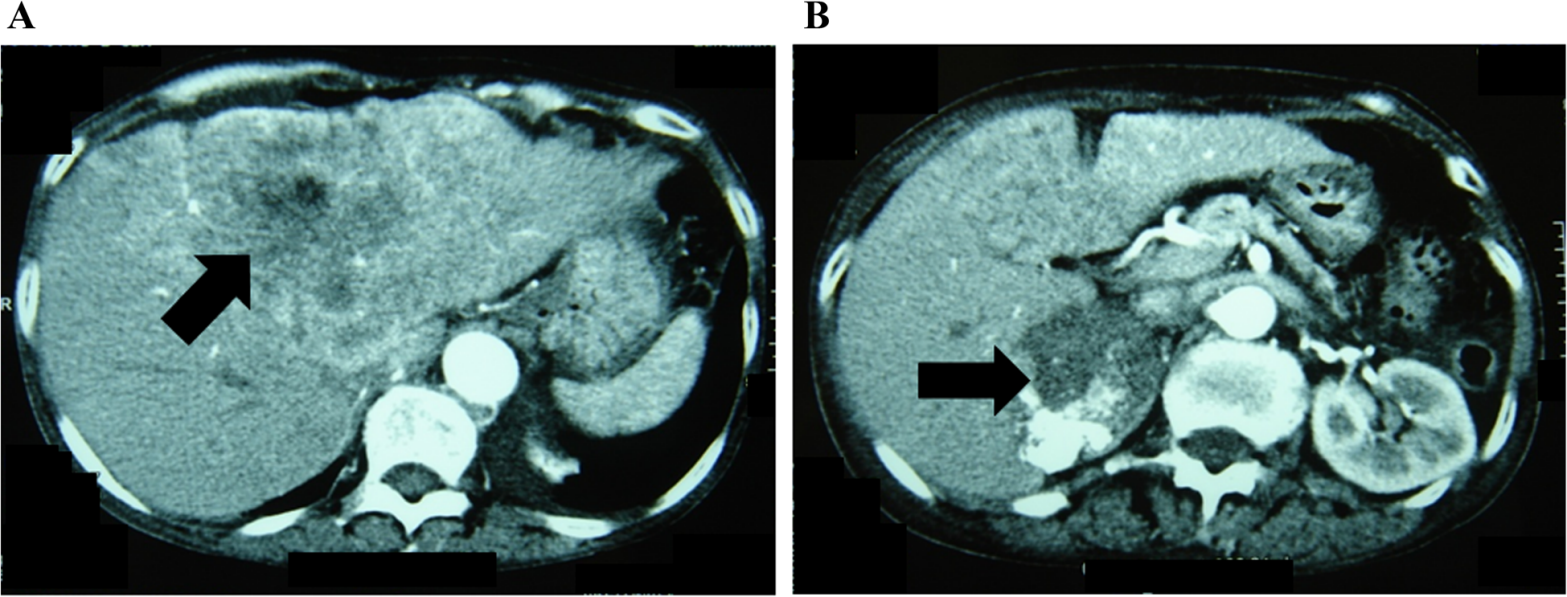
Abdominal dynamic computed tomography. Abdominal dynamic computed tomography shows a huge liver tumor (14 cm in diameter) and right adrenal tumor (6 cm in diameter). a slice of liver tumor; b slice of right adrenal tumor
